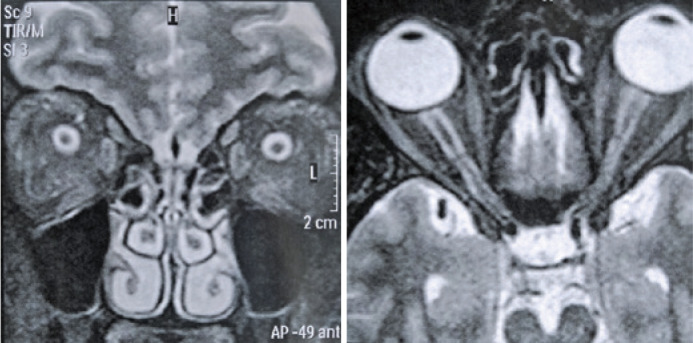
MRI of the brain and the orbits showing flattening of the globe, an
